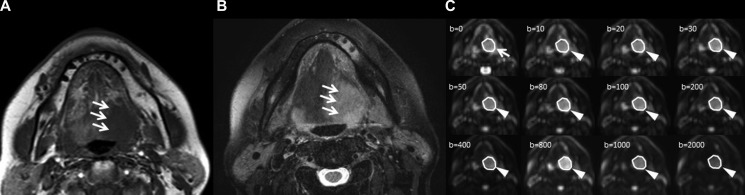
Tumor ROI delineation A patient whose primary tumor was in the base of the tongue is presented. With the depiction of the primary tumor in T1WI (A; arrow) and T2WI (B; arrow) as a reference for the ROI delineation, the primary tumor was outlined by a polygonal ROI on b0 images of DWI (C; arrow), and the tumor ROI was then copied on the EPI of the respective b-values (c; arrowhead). The original images are presented with the same window level/width in the range of 0–400 of b-value, whereas each window level/width was adjusted for good visualization of the tumor for the b-values ≥ 800.
